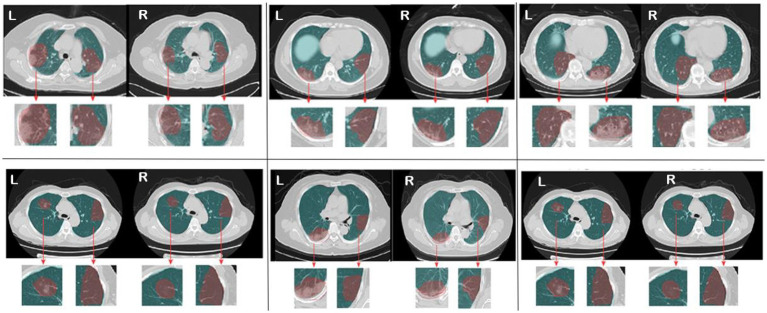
Pre- and post-treatment chest CT scans of a patient diagnosed with COVID-19, highlighting pneumonia lesions (red circles). The pre-treatment scan (L) shows initial lung involvement, while the post-treatment scan (R) demonstrates changes following medical intervention. This comparison helps assess treatment effectiveness and the resolution of pneumonia-related abnormalities.
